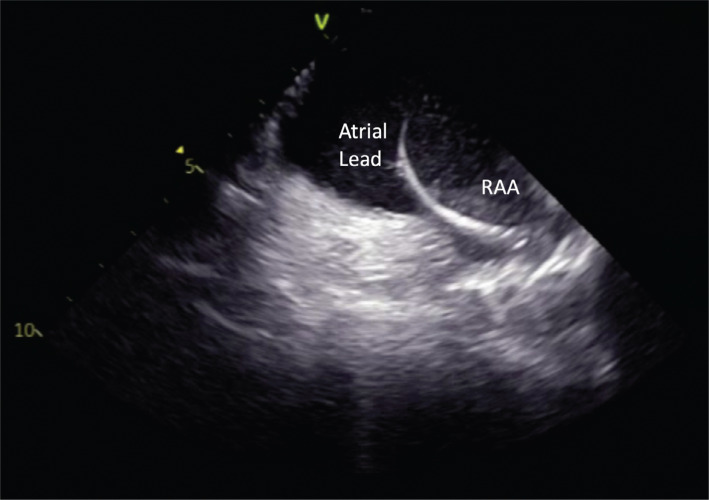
Intracardiac echocardiography image showing an atrial lead in the right atrial appendage. *Abbreviation:* RAA, right atrial appendage.
